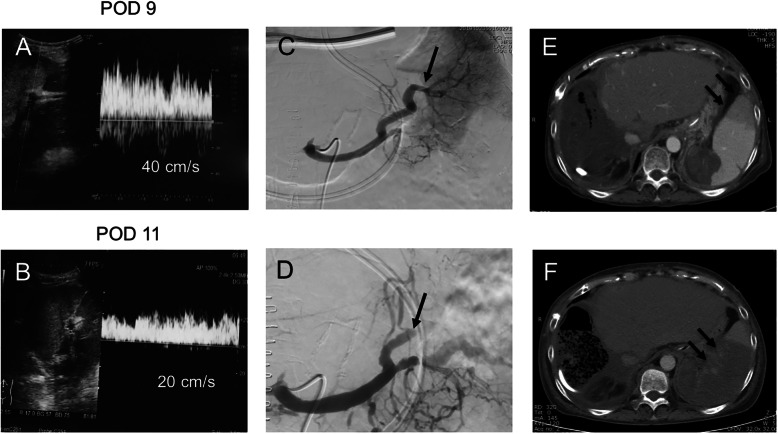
Postoperative assessment of portal flow and dynamic CT image before (a, c, e) and after (b, d, f) splenic artery embolization. a Portal flow at the umbilical portion on postoperative day (POD) 9. b Portal flow on POD 11 at the same branch of the portal vein, which had decreased to half of that on POD 9. c Splenic arterial overflow with splenomegaly showed enhanced flow on injection of the contrast. Arrow, the main branch of the splenic artery. d The main branch of the splenic artery was completely occluded by trans-catheter coil embolization on POD 10. Arrow, the main branch of the splenic artery. e Dynamic computed tomography (CT) before splenic artery embolization showed only the upper pole of the spleen turns pale by the ligation. Arrow, paled lesion of the spleen. The lower pole has lost the arterial flow due to previous partial splenic artery embolization. f Dynamic CT after splenic artery embolization showed most of the spleen lost the arterial flow. Arrow, paled lesion of the spleen. POD, postoperative day; CT, computed tomography
